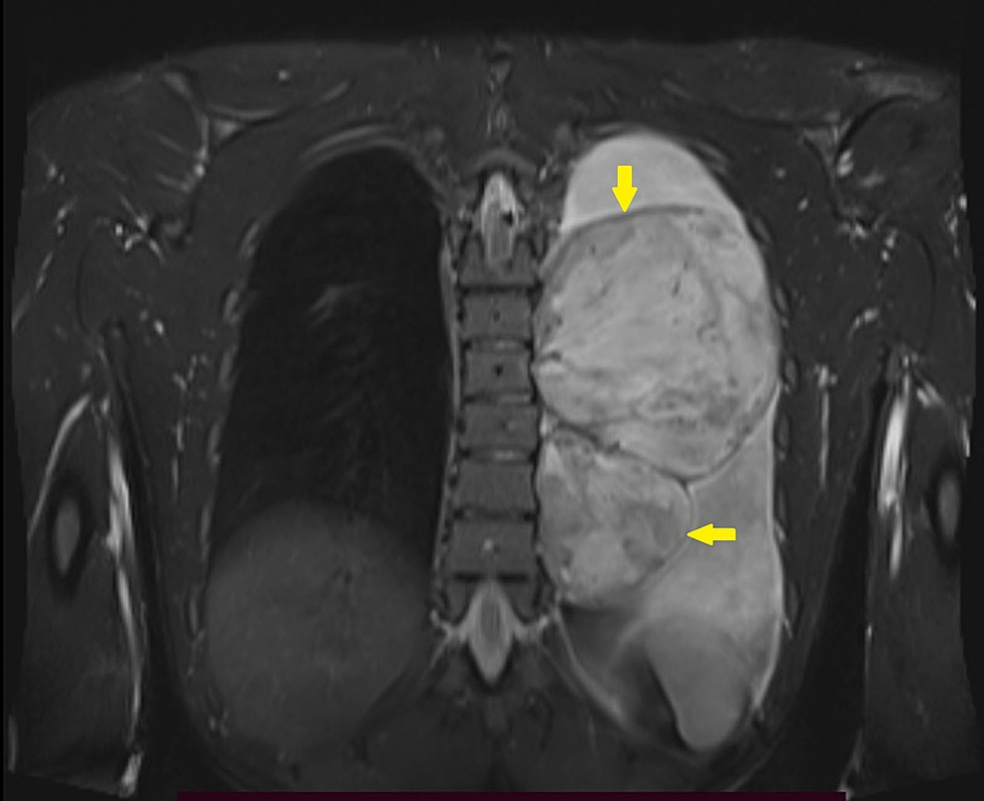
Coronal section of T2 weighted MRI shows two T2 hyperintense pleural-based lesions (yellow arrows) within the left pleural cavity with associated pleural effusion
